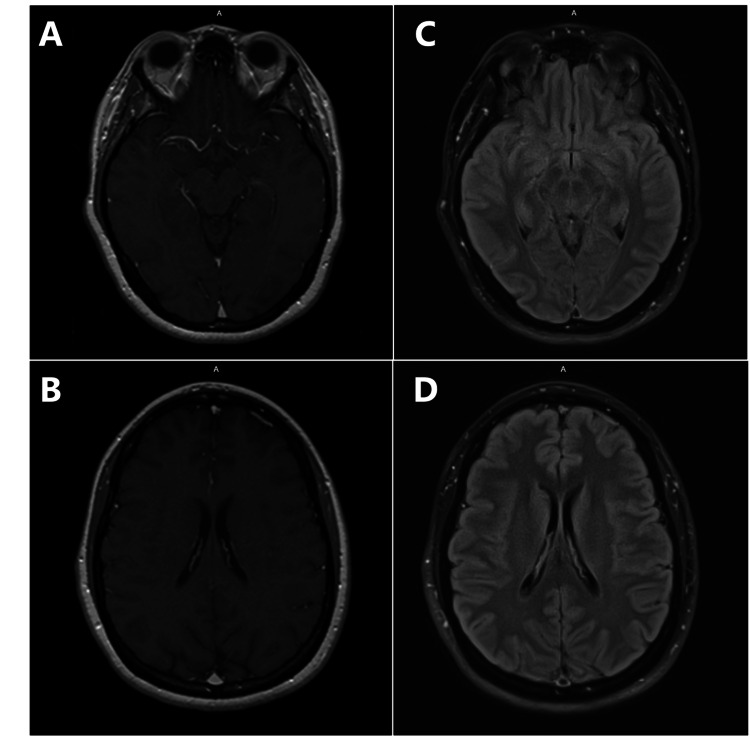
Radiological findings of the brain. Magnetic resonance imaging on T1 (A and B) and fluid-attenuated inversion recovery (FLAIR) (C and D) showed no acute infarct, hemorrhage, or mass lesion. The ventricles, cisterns, and sulci were not effaced.
